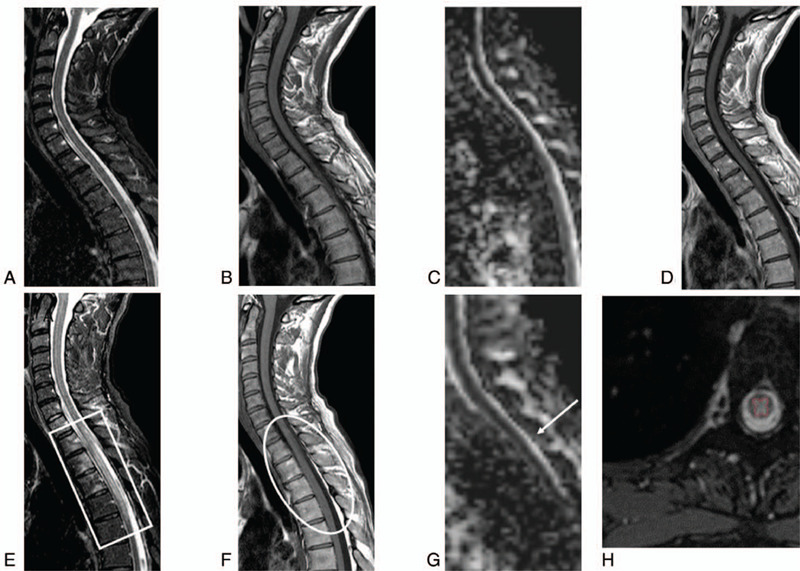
MRI of the spinal cord at 9 and 72 h. MRI images: in the acute phase (A, B, C, D sagittal images; respectively STIR T2, TSE T1, ADC map and TSE T1 post-gad) no spinal cord alterations were seen; the exam was acquired 9 h from the clinical onset. Seventy-two hours later, a new MRI scan (respectively E, F, G sagittal images STIR T2, TSE T1, and ADC map and H axial TSE T2) detected a spinal cord lesion extending from T1-T2 to T6 with a bulgy appearance of the spinal cord (white circle) and a hyperintense signal on STIR T2 images (white rectangle). Moreover, a mild restriction of diffusivity on ADC map, compared to the previous exam (white arrow) and a signal alteration of some vertebral bodies from C7 to T4, more obvious on STIR T2 images as bone marrow edema can be noticed. Axial TSE T2 images (H) showing the prominent gray matter involvement (red line) is suggestive of ischemia rather than myelitis. MRI = magnetic resonance imaging.
